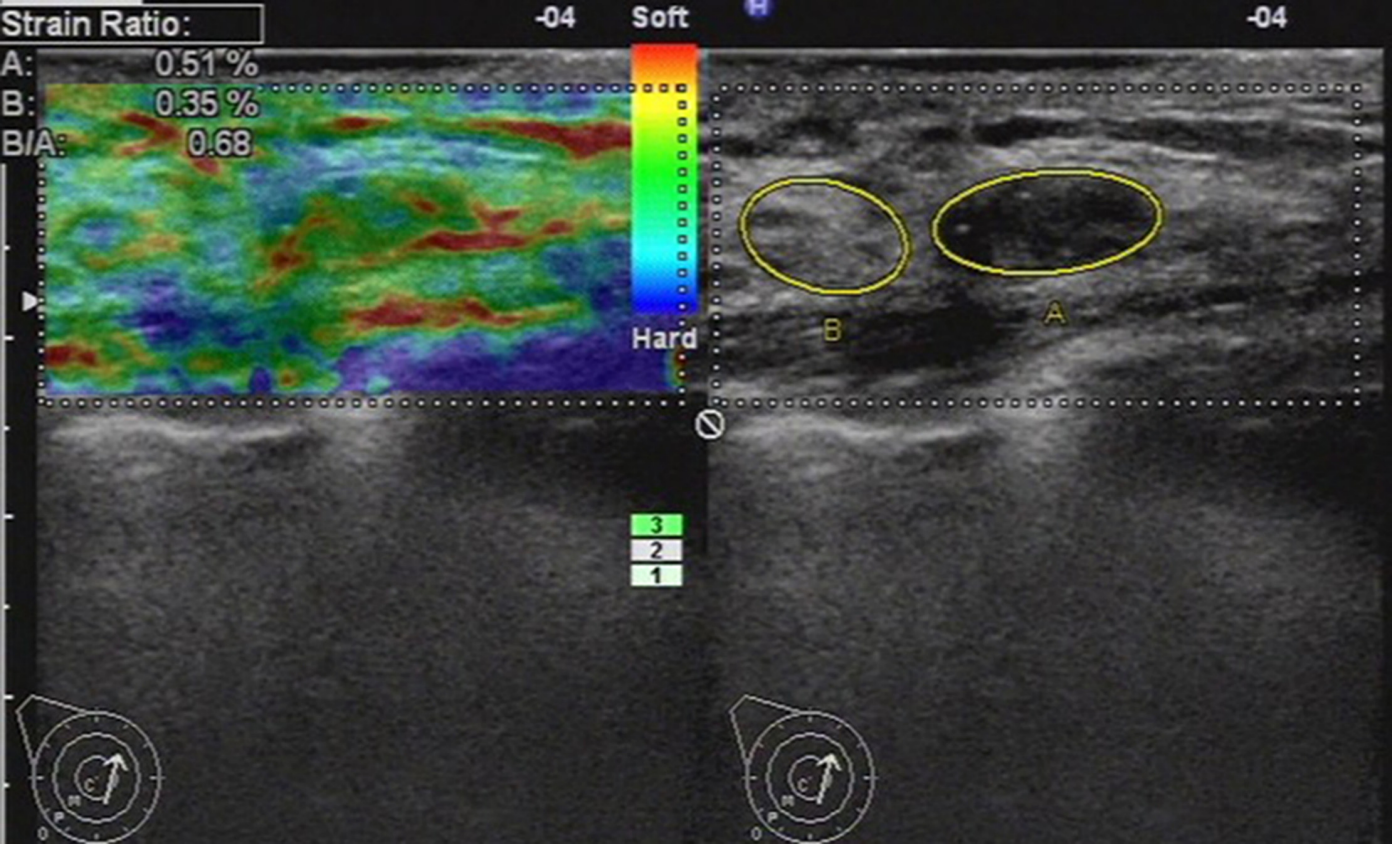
Ultrasonic elastographic image of a fibroadenomatous hyperplasia in a 39-year-old woman. The lesion was scored 1 with the 5-point scoring method. The strain ratio was 0.68 with the strain ratio method at the same breast tissue depth as the reference.
